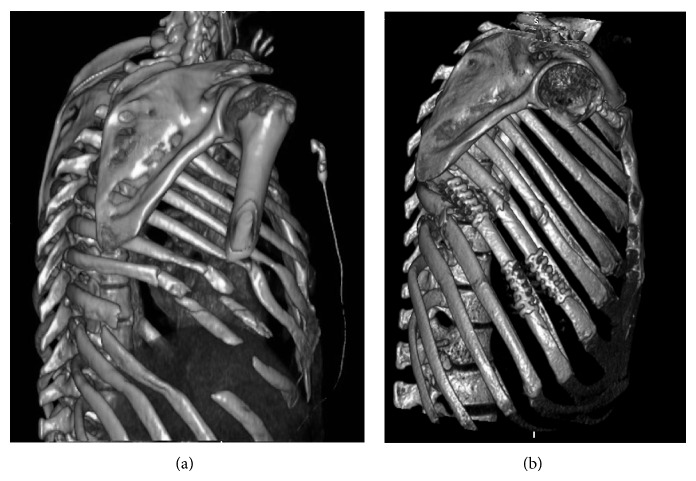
3D-computed tomography bony thorax reconstruction (a) demonstrating flail chest and facilitating the surgical approach and (b) demonstrating flail chest reconstruction, with good cosmetic and functional results.
